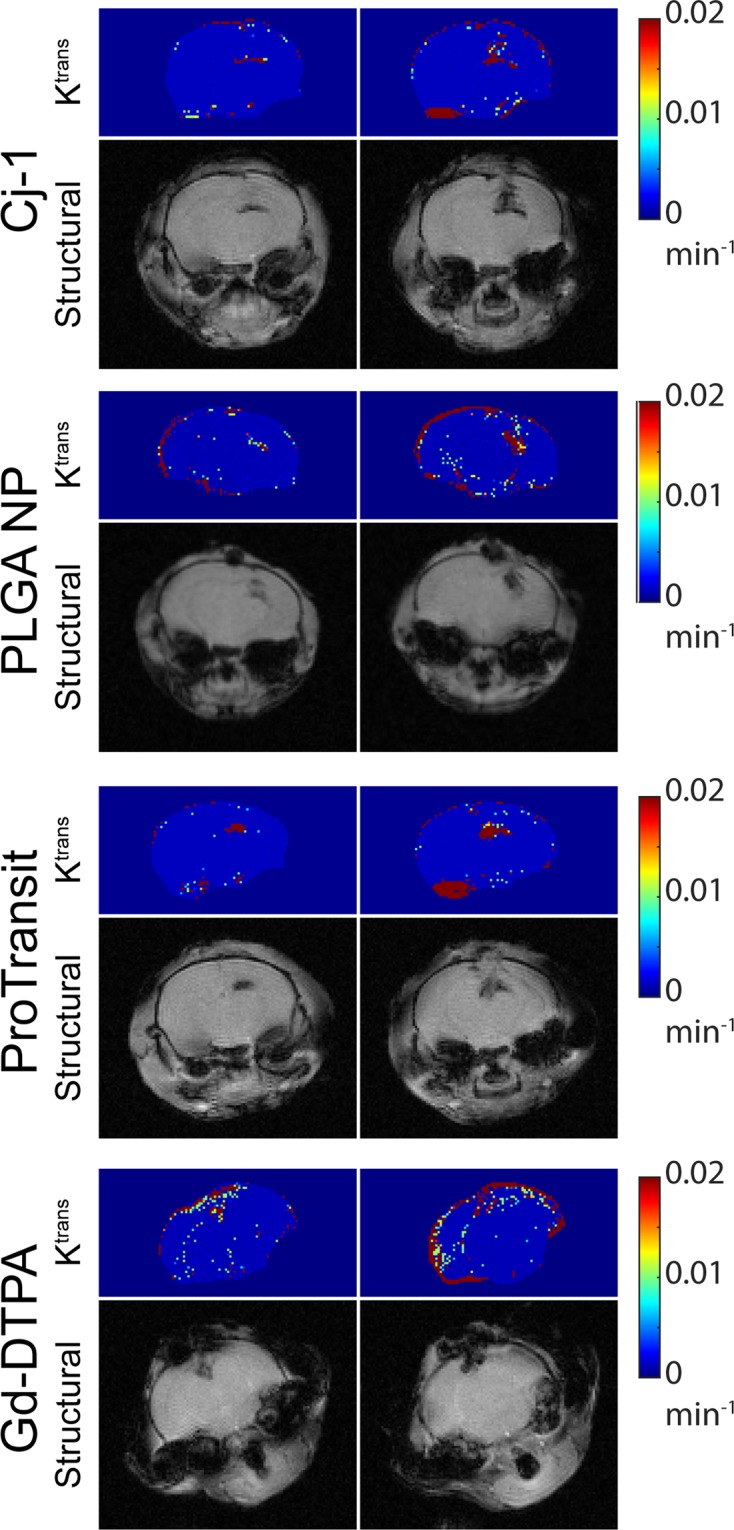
K^trans^ maps and corresponding structural MR images for all NP types. Structural images provide information on the injury location in the brain. Maximum values in K^trans^ maps (dark red) correspond to injury shown in the structural images (darker brain regions). This correspondence indicates areas of highest uptake are damaged tissue.
